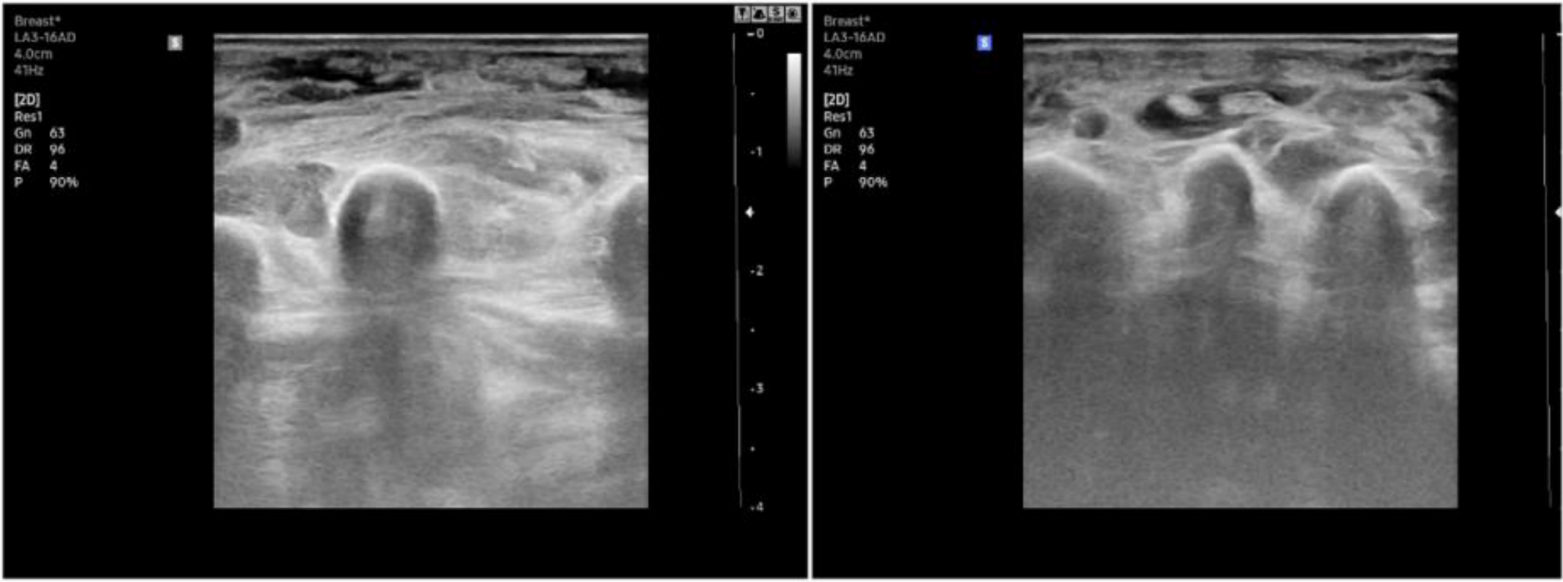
Swelling of soft tissue and peritendinous effusion in hand ultrasounds.
